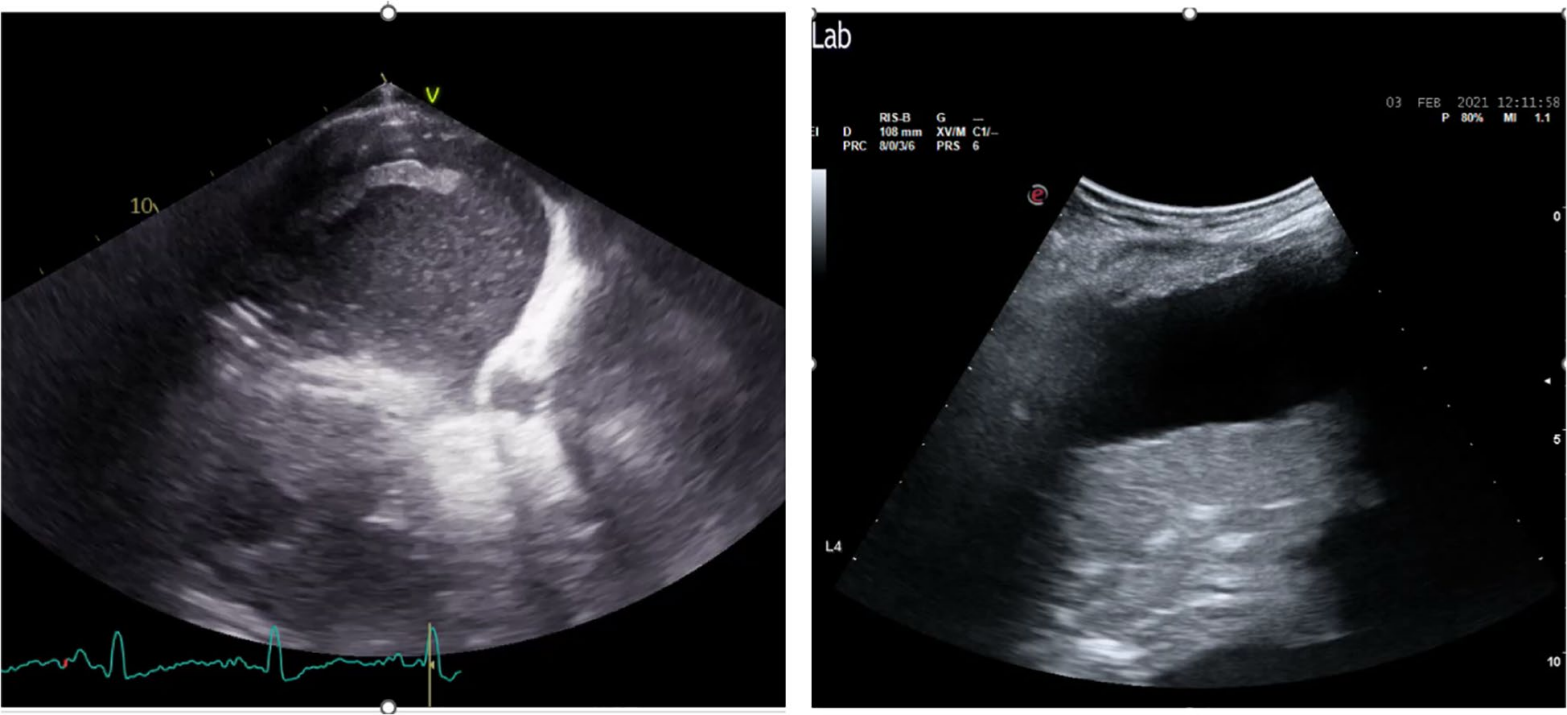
Lung Ultrasound (LUS) finding: pleural effusion characterized by exudate on the left panel and transudate on the right panel
